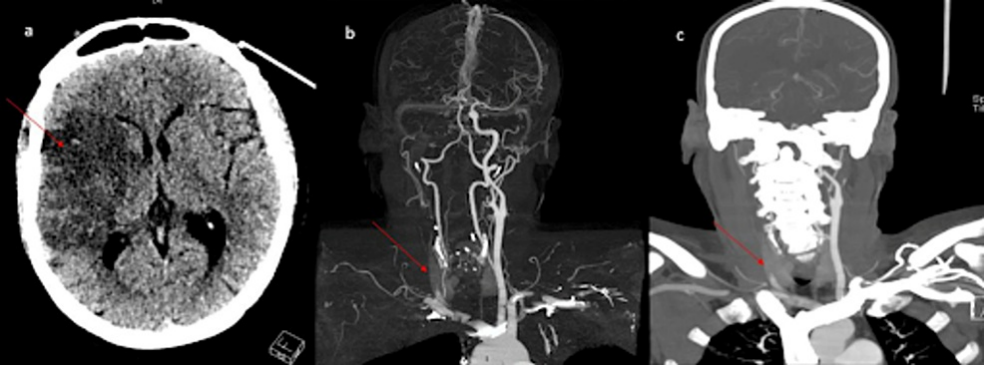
Non-contrast CT scan head and cerebral angiogram showing right side large vessel middle cerebral artery ischemic stroke of a COVID-19 patient. Non-contrast CT scan of the head shows a large vessel ischemic infarct in the right middle cerebral artery territory (a). CT cerebral angiogram and MIP images (as shown in b, c) show complete occlusion of right common carotid artery starting from its proximal end with non-visualization of external common carotid, internal carotid, and middle cerebral artery (b, c).
